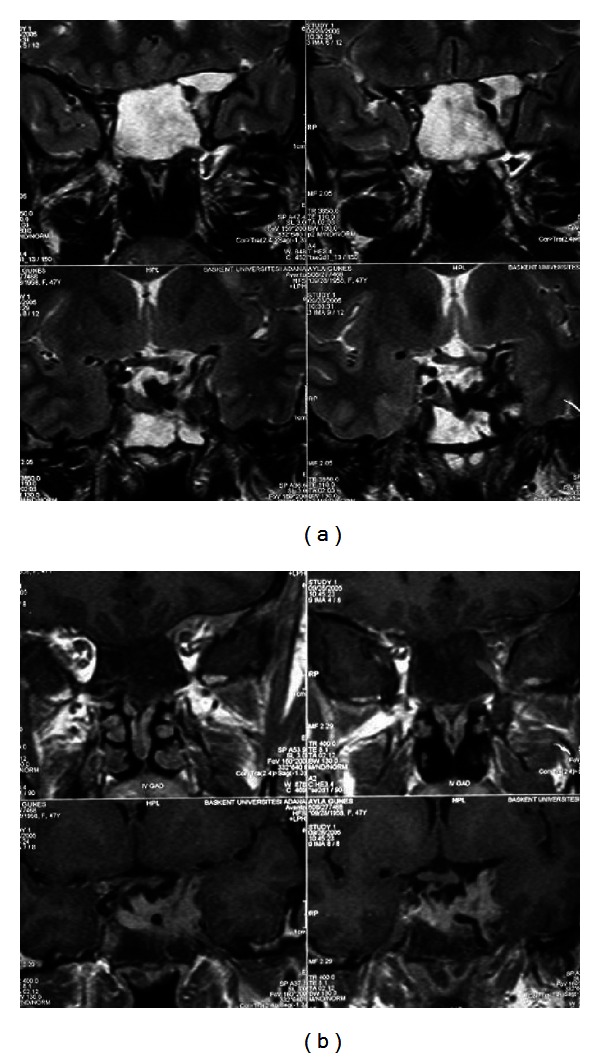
Follow-up magnetic resonance imaging, (a) coronal T2-weighted turbo spin echo image shows cyst formation within the macroadenoma with a small solid portion on the left lateral side. (b) Postcontrast coronal spin echo image revealed resolution of the macroadenoma in the suprasellar cistern.
